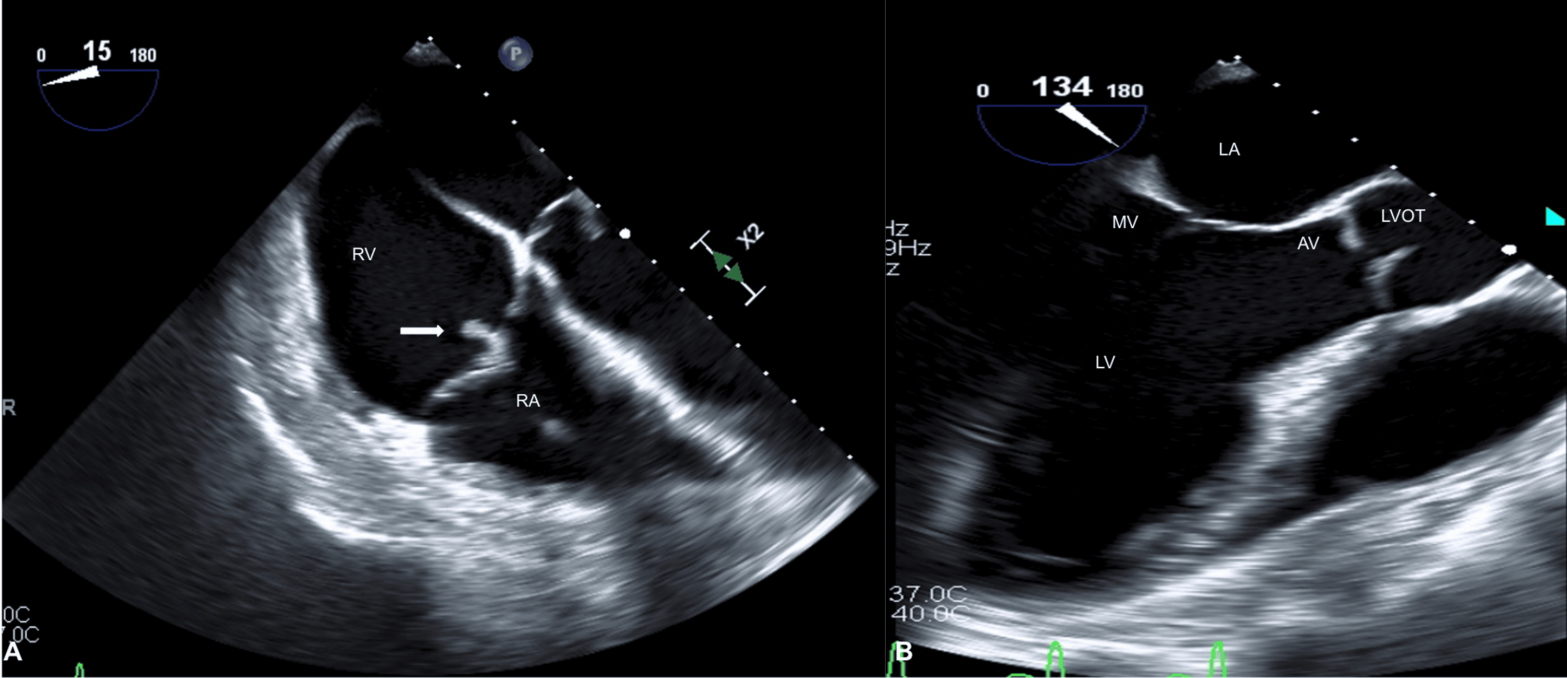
Transesophageal echocardiogram showing A) mobile echodensity on tricuspid valve measuring 0.7 x 0.7 cm (white arrow); B) normal mitral valve and aortic valve without vegetations RV: right ventricle, RA: right atrium, LA: left atrium, MV: mitral valve, LV: left ventricle, AV: aortic valve, LVOT: left ventricular outflow tract
